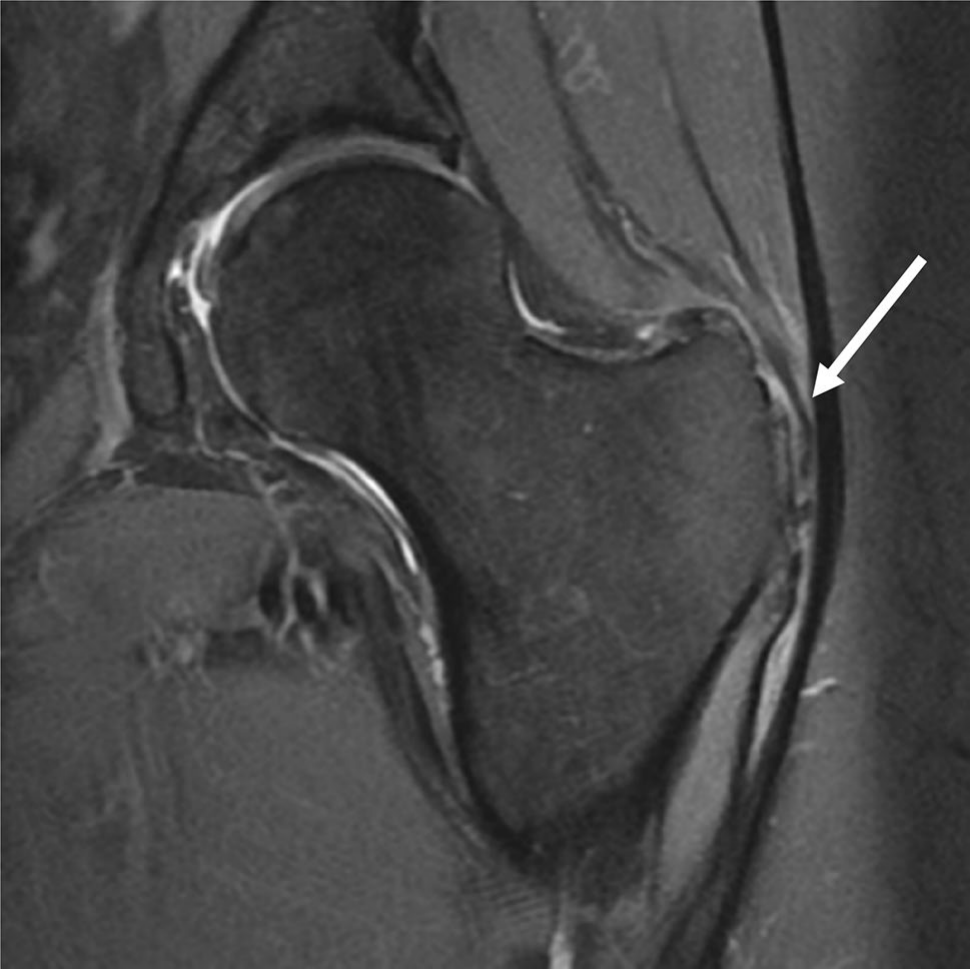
MRI T2 sequence showing hyperintensity around the greater trochanter, signifying GTPS
